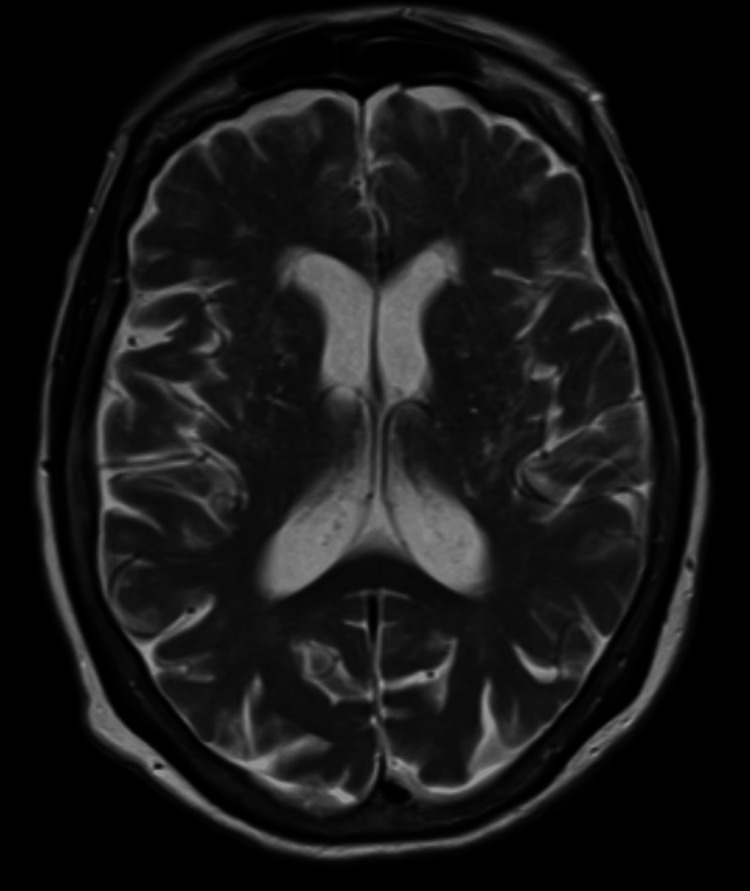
An MRI of the brain shows no acute abnormality
